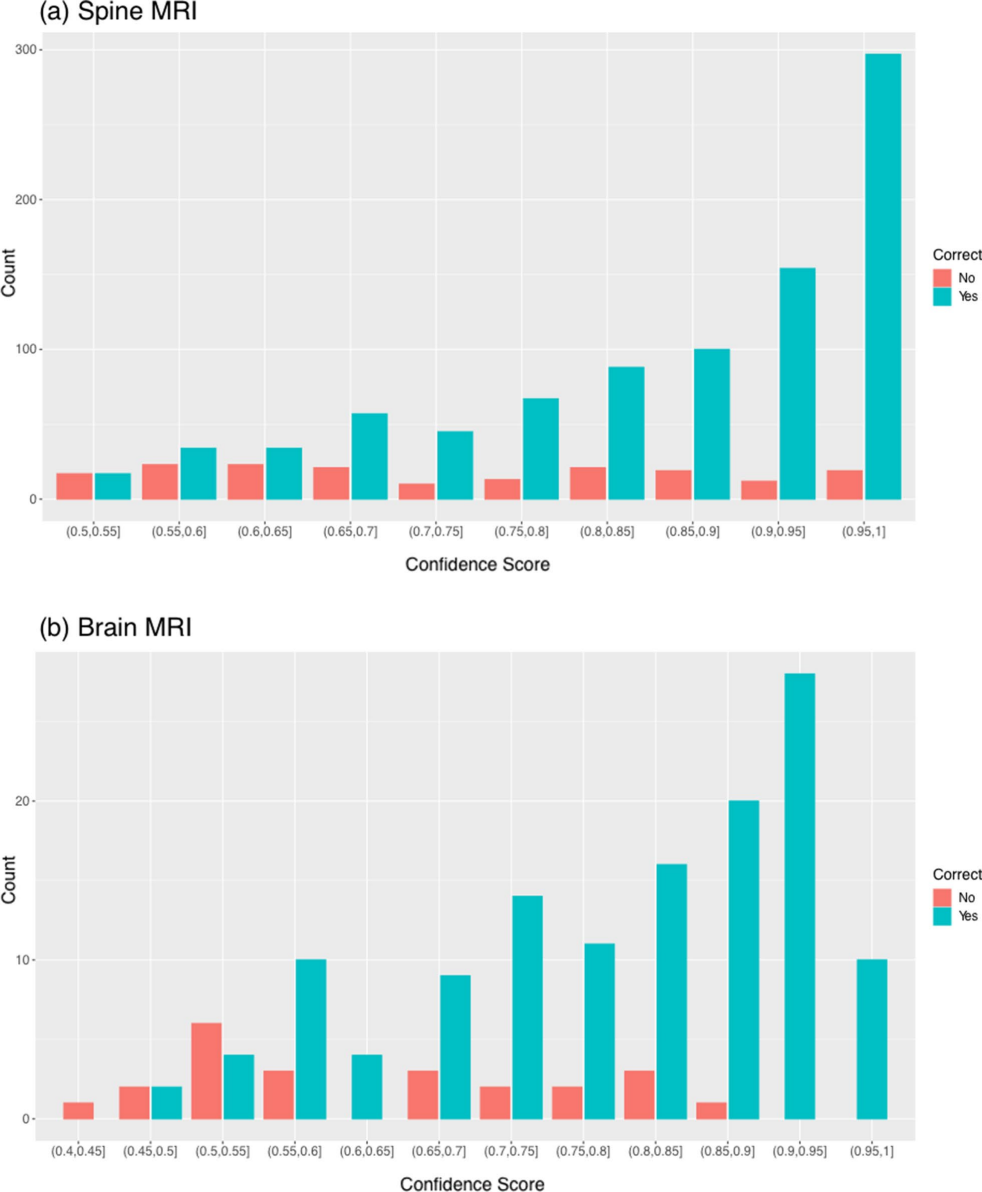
Confidence score of correct and incorrect predictions. A histogram of confidence scores for correct and incorrect protocol predictions. Confidence score obtained from model output on scale of 0–1. a For spine MRI prediction, confidence score distribution of incorrect predictions differed significantly from correct predictions (Kolmogorov–Smirnov test, *p* < 0.001). Predictions were skewed towards high confidence, while incorrect predictions were more uniformly distributed. b For brain MRI predictions, confidence score distribution of incorrect predictions differed significantly from correct predictions (Kolmogorov–Smirnov test, *p* < 0.001). Correct predictions were skewed towards high confidence
